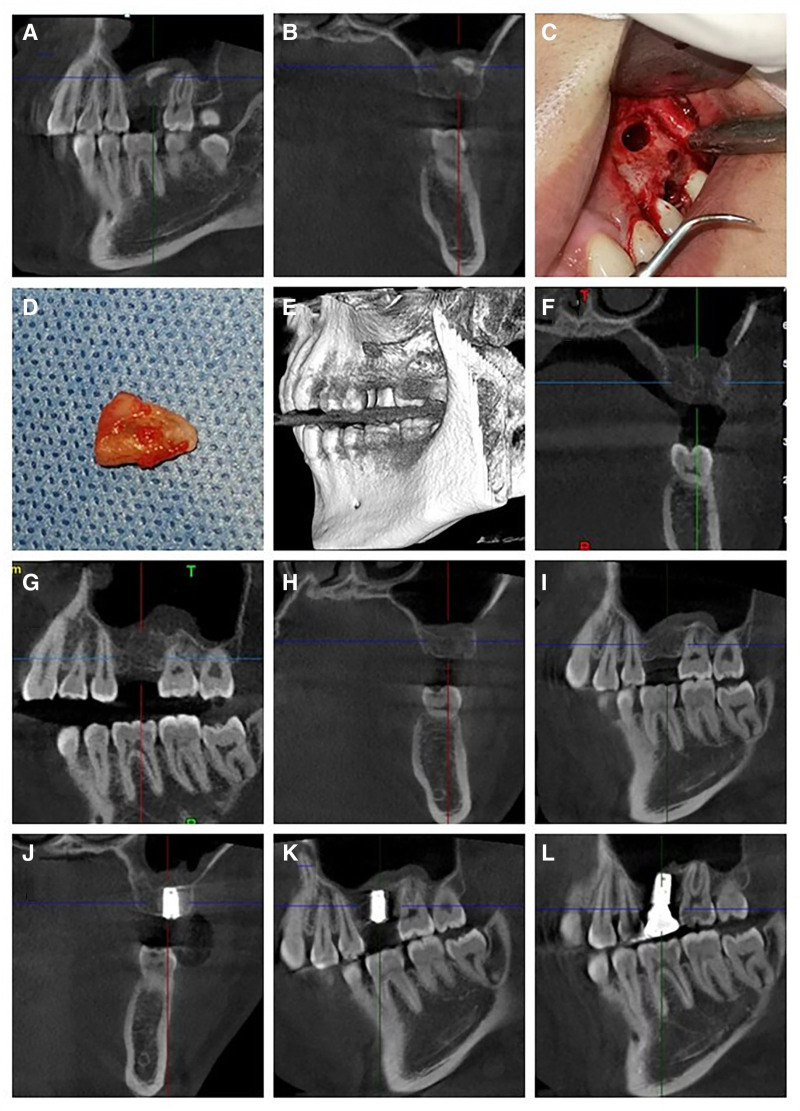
CBCT images, intraoral photographs, and the extracted root stump of case 2. (A and B) CBCT images showing the root stump within the left maxillary sinus. (C) Elevation and separation of the maxillary sinus membrane following lateral window opening on the left maxillary sinus. (D) The extracted root stump. (E) Three-dimensional reconstruction showing the bone window on the lateral wall of the left maxillary sinus. (F and G) Postoperative CBCT images showing no foreign objects within the left maxillary sinus, with no significant effusion. (H and I) Good healing of the extraction site at position 26, 4 months postoperatively. (J and K) Routine implant placement at site 26, 4 months postoperatively. (L) Completion of implant restoration at site 26. CBCT = cone-beam computed tomography.
